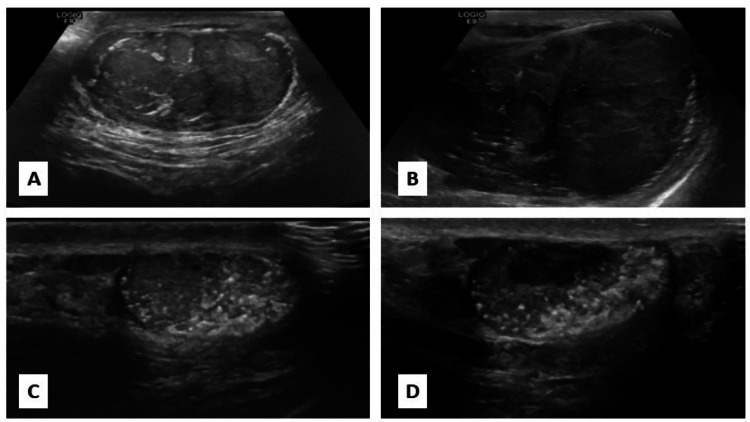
Ultrasound images showing development of new bilateral testicular masses. Ultrasound of the left testis showing the development of a new heterogeneous mass (B) that was not evident in the previous ultrasound (A). Ultrasound of the right testis showing a small testis (C) with the development of a small heterogeneous mass in the new ultrasound, without any change in testis size (D).
